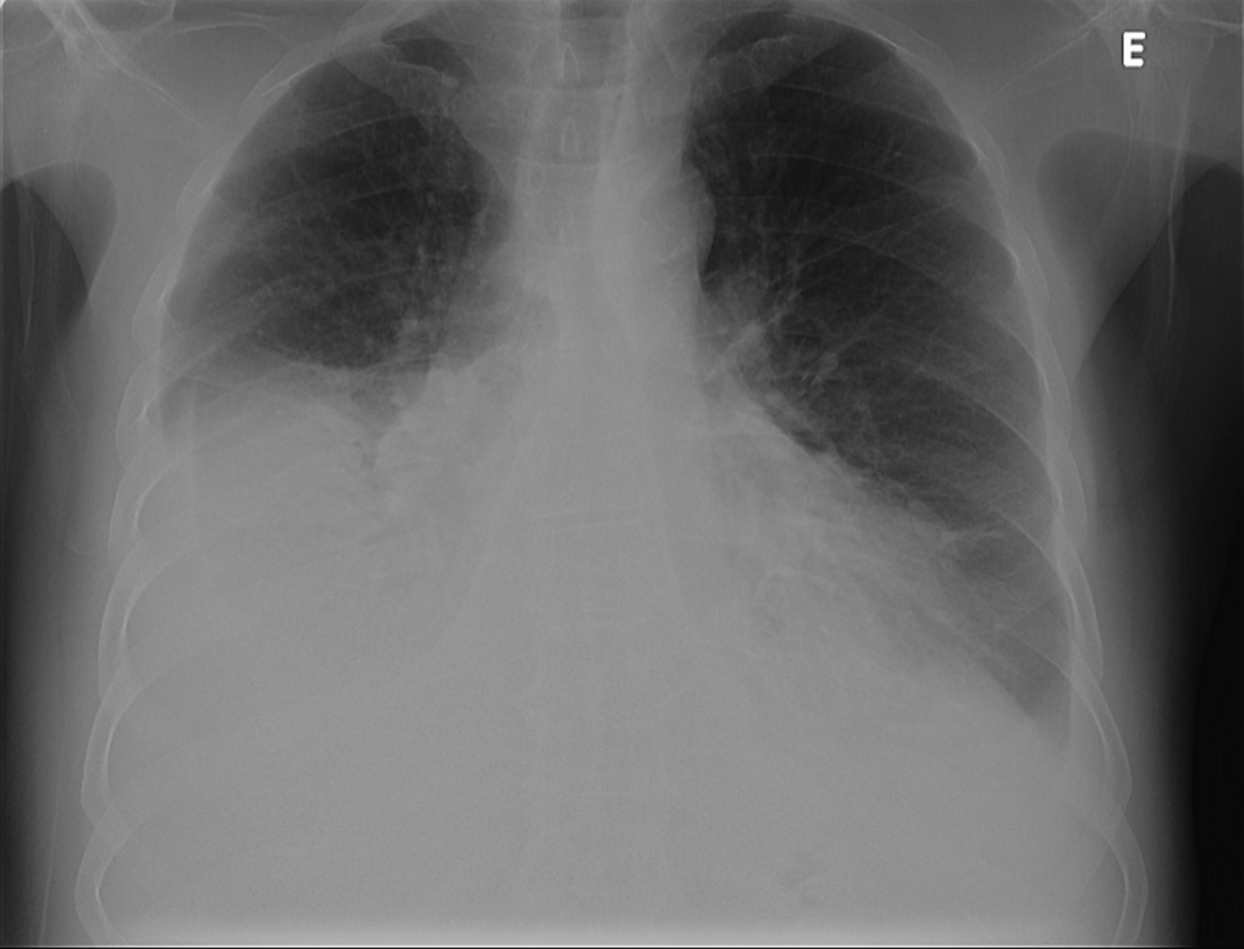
Chest radiography, posteroanterior view Chest radiography, posteroanterior view, on admission, showing voluminous right pleural effusion.
